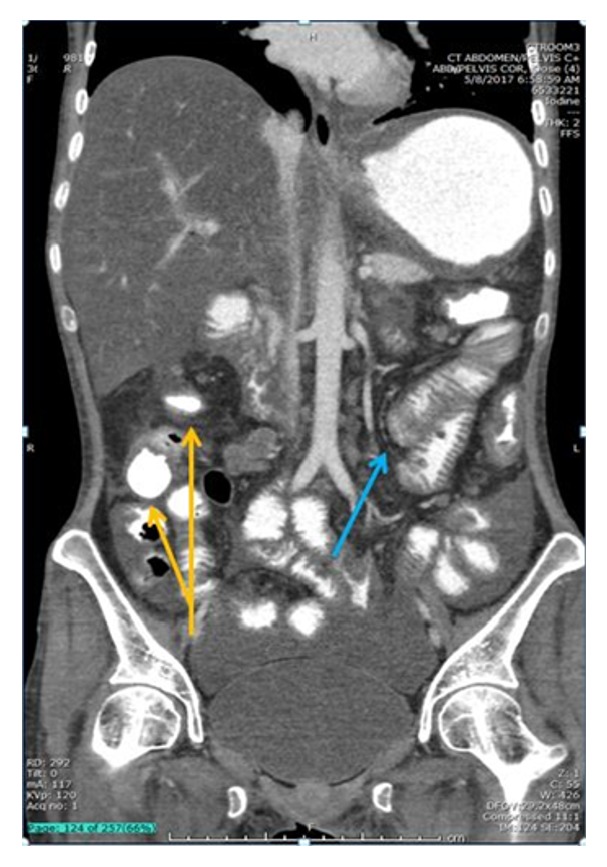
CT demonstrating concentric bowel wall thickening in the region of the splenic flexure (blue arrow) with sparing of the region of the hepatic flexure (yellow arrow). The patient later developed colonic obstruction at both sites.
